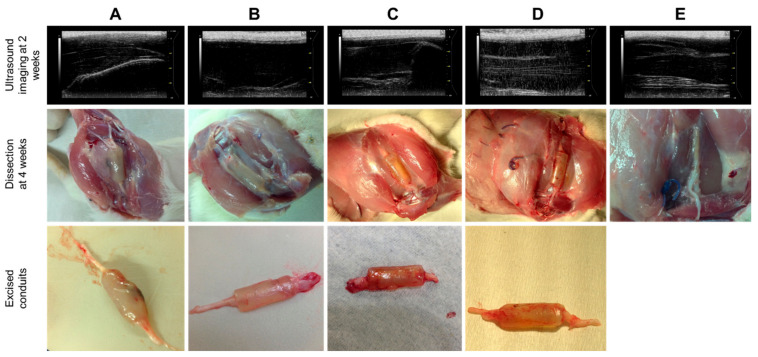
Images of ultrasound analysis, post-implantation and excised conduit of Groups (A–E).
